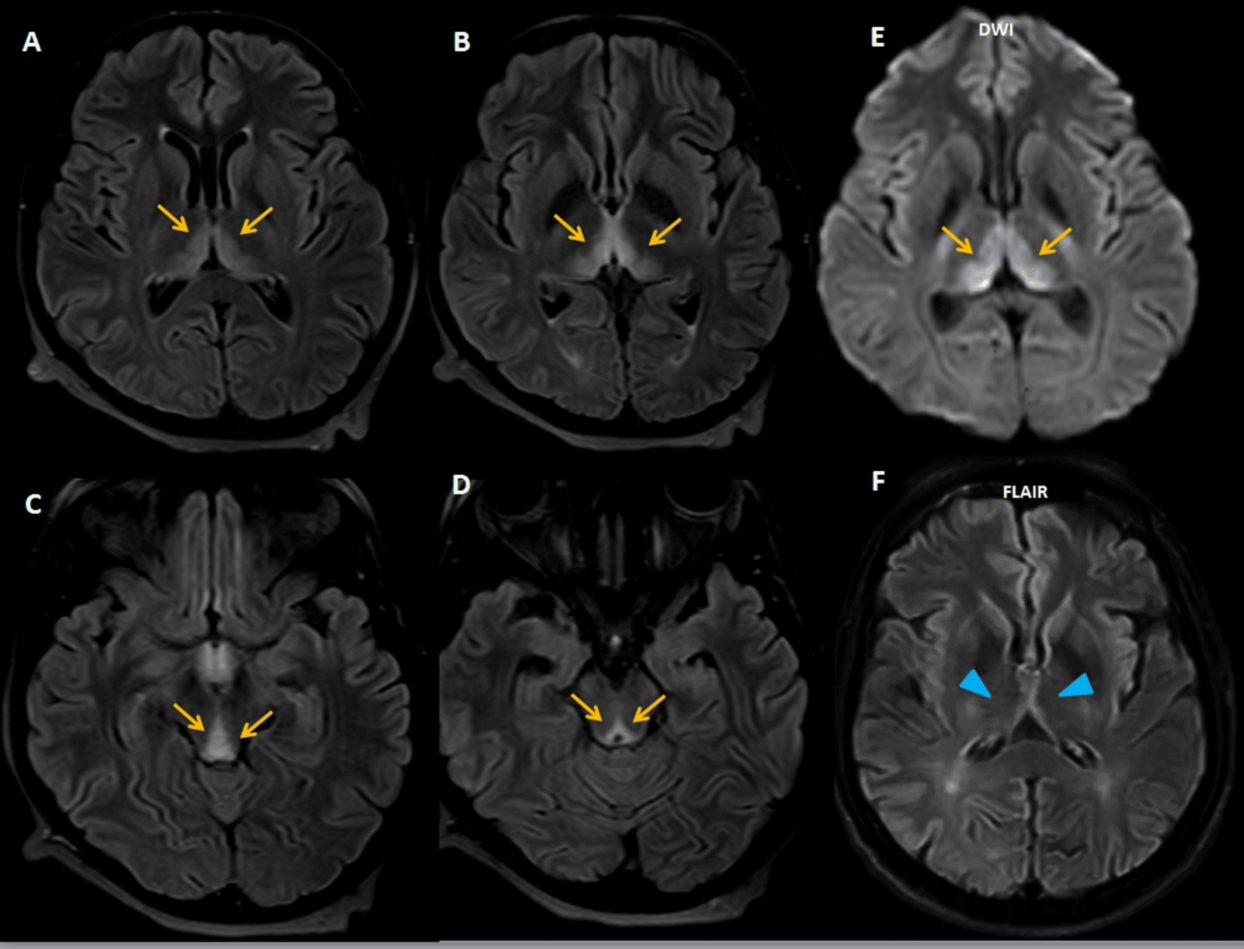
MRI of the brain of a 24-year-old pregnant woman (G3A2, 14 weeks gestation) with a history of persistent vomiting for 15 days presented with limb weakness and slurred speech. (A-D) Axial FLAIR images demonstrate symmetrical hyperintensities (orange arrows) in the dorsomedial thalami, around the third ventricle, periaqueductal gray matter, and tectal plate, typical of acute WE secondary to thiamine depletion resulting from hyperemesis gravidarum. (E) Additional DWI showing corresponding diffusion restriction. (F) Follow-up MRI after thiamine supplementation shows resolution of signal changes, indicating radiological recovery (blue arrowhead). She delivered a healthy baby via elective cesarean at 39 weeks MRI: magnetic resonance imaging, FLAIR: fluid attenuated inversion recovery, WE: Wernicke’s encephalopathy, DWI: diffusion-weighted imaging
